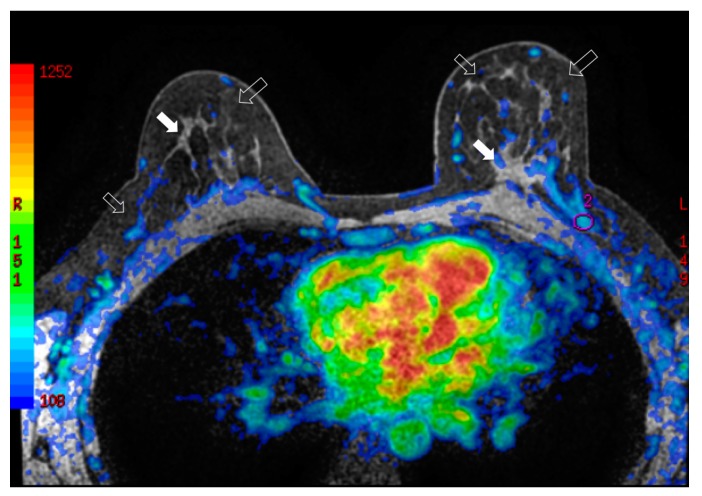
Bilateral three-dimensional MRI T1-weighted spoiled turbo gradient echo image after contrast media administration (colored superimposed image). Using a special fat saturation and separate shim volumes on each breast, VIBRANT sequence (Volume Imaging for Breast Assessment) allows axial acquisition with an excellent separation of glandular tissue (white arrows) from fat tissue (white empty arrows). The velvet region of interest shows how the vascularity of the left breast compared to the contralateral breast after fat grafting is increased.
